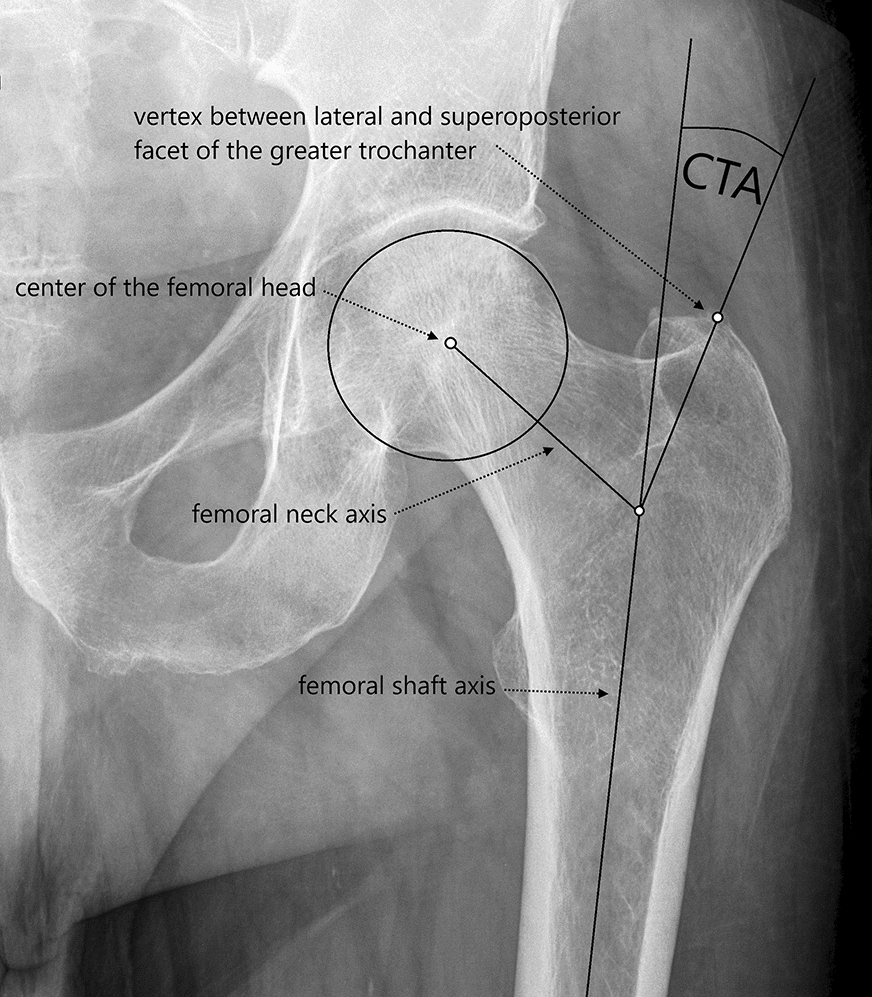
Measurement of the ‘critical trochanter angle’ (CTA) as described by Haversath et al. [11]
